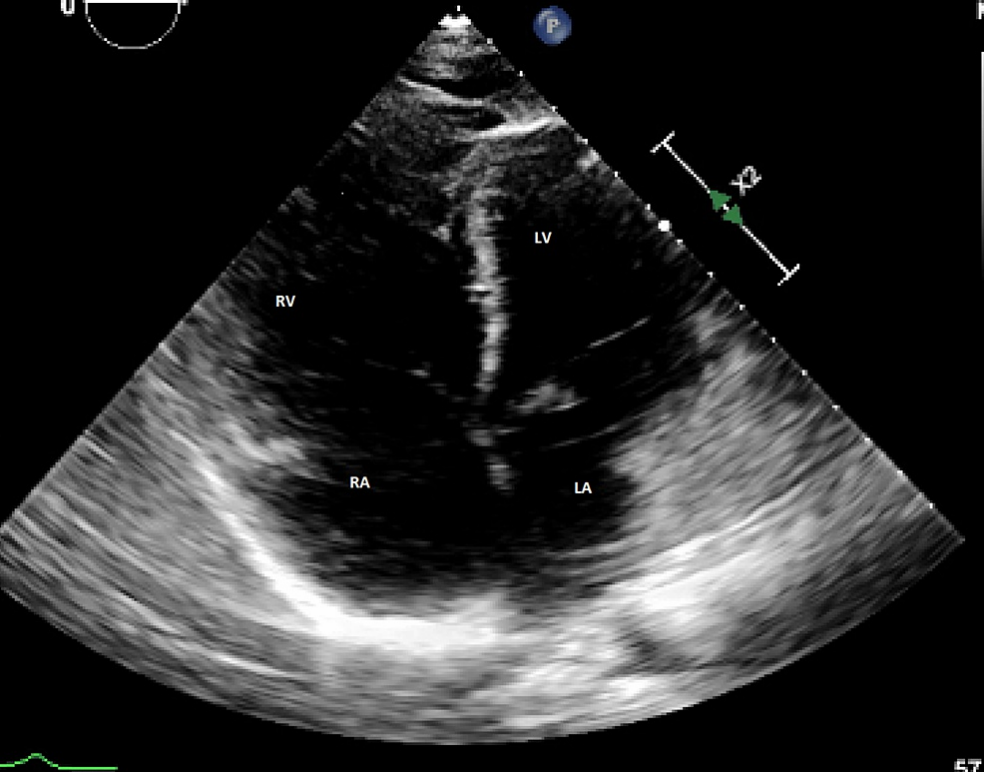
Transthoracic echocardiogram with apical four-view with severely dilated right ventricle and severely reduced right ventricular global systolic function and flattening of intraventricular septum seen during systole RV: right ventricle; RA: right atrium; LV: left ventricle; LA: left atrium
